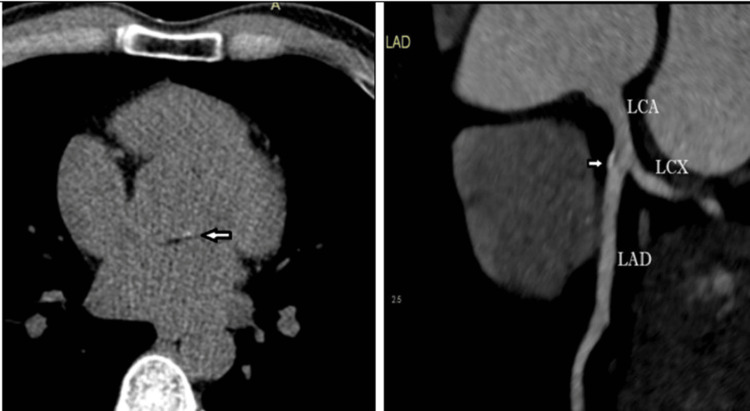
Plain images of CT showing (left) mild calcification at the aortic root (arrow). CT Coronary angiography image (right) showing mixed density plaque in ostioproximal LAD causing no significant stenosis. LCA: left coronary artery; LAD: left anterior descending artery; LCX: left circumflex artery
